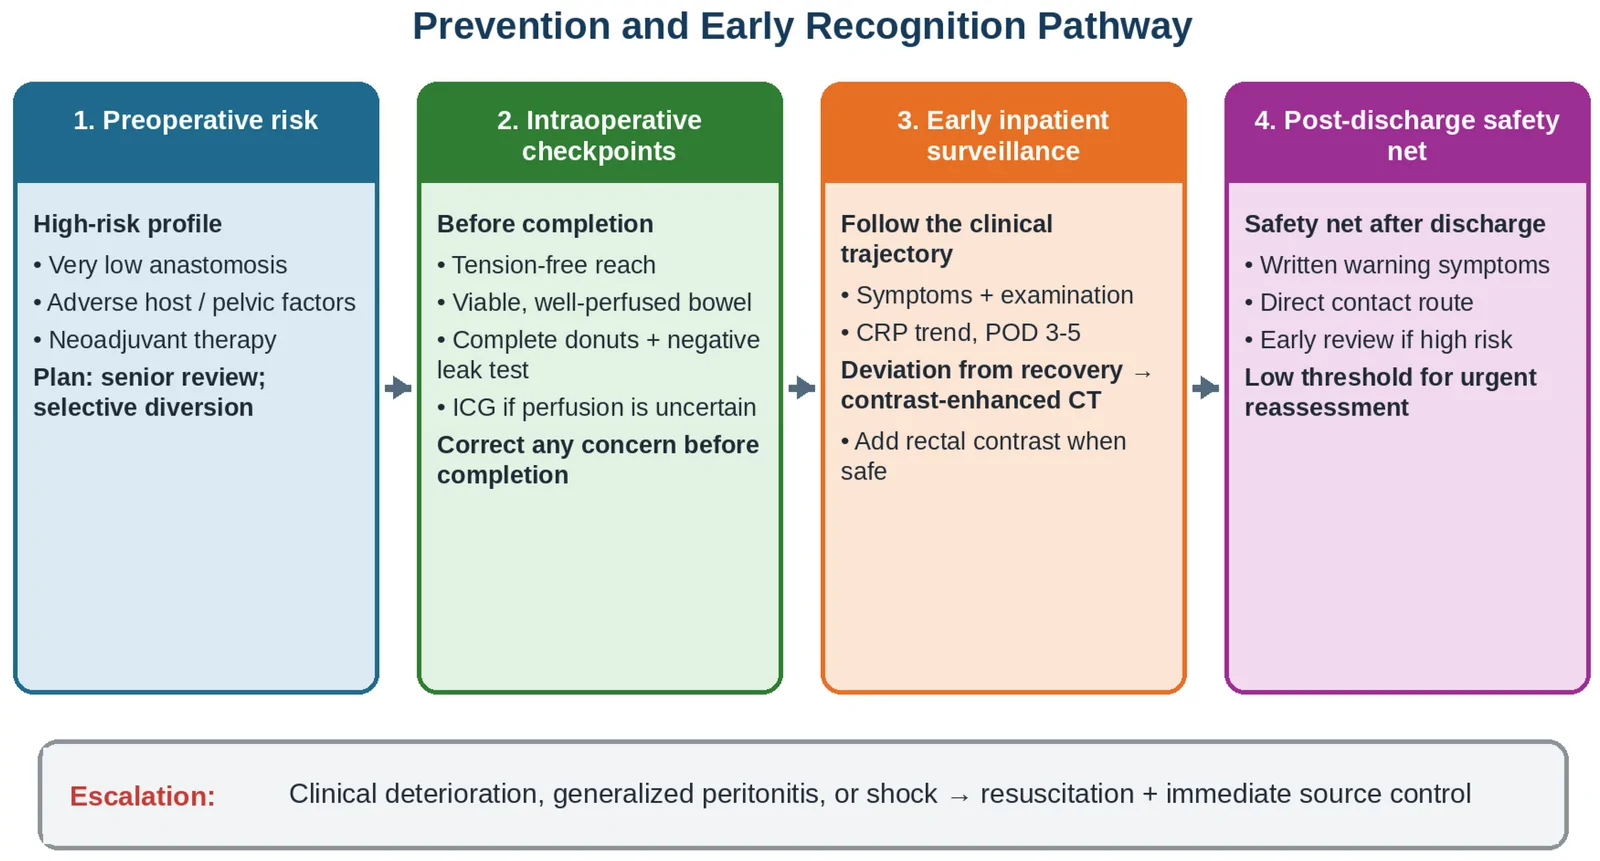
Prevention-to-recognition pathway for AL after rectal cancer surgery. Examples of early warning features include fever, tachycardia, pelvic pain, ileus, urinary symptoms, abnormal drain output, poor appetite, fatigue, pelvic pressure, and low-grade fever. Approximate CRP rule-out ranges from pooled colorectal data are POD 3, 148–172 mg/L; POD 4, 123–124 mg/L; and POD 5, 115–144 mg/L [49,50]. These values should be interpreted in clinical context and do not replace clinical judgment. High-risk patients require a low threshold for reassessment during the first 2 postoperative weeks. Abbreviations: CT, computed tomography; ICG, indocyanine green; POD, postoperative day. The colors distinguish pathway stages rather than quantitative risk levels.
